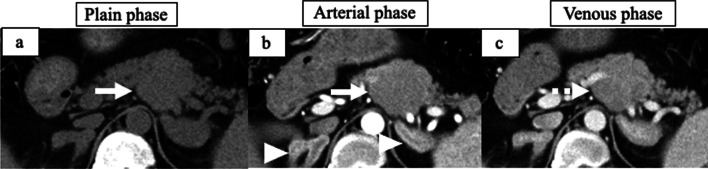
Enhanced CT imaging. a Plain CT reveals the tumor located in the pancreatic tail (55 mm in diameter; white arrow). b Enhanced CT reveals the tumor with enhancement (white arrow) and bilateral adrenal enlargement (white arrowhead). c Enhanced CT reveals the tumor persisting with weak enhancement (dotted white arrow). *CT* computed tomography
